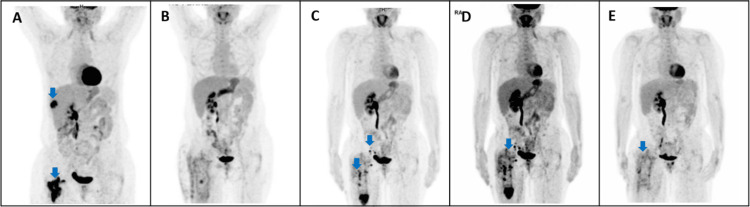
Disease evolution on 18fluorodeoxyglucose positron emission tomography (18FDG-PET) images A) Blue arrows demonstrate liver and right femur metastases (December 2020); B) Absence of 18FDG uptake, indicating complete response (November 2021); C) Blue arrows demonstrate right femur and ipsilateral inguinal and external iliac lymph nodes metastases (October 2022); D) Worsening 18FDG uptake of the right femur and ipsilateral inguinal and external iliac lymph nodes (January 2023); E) Minor 18FDG uptake by right hip indicating disease persistence or inflammatory disease (September 2023)
